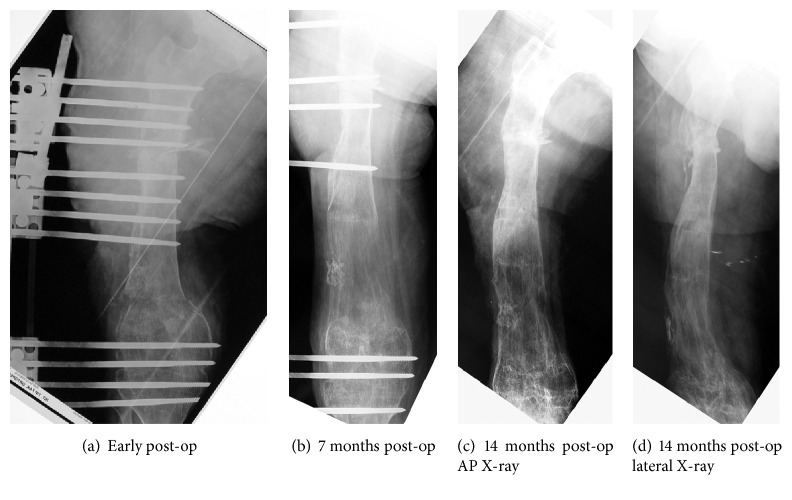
(a) Postoperative radiography of the femur. (b) Post-op 7-month radiography of the femur. (c, d) Post-op 14-month AP and lateral radiography.
